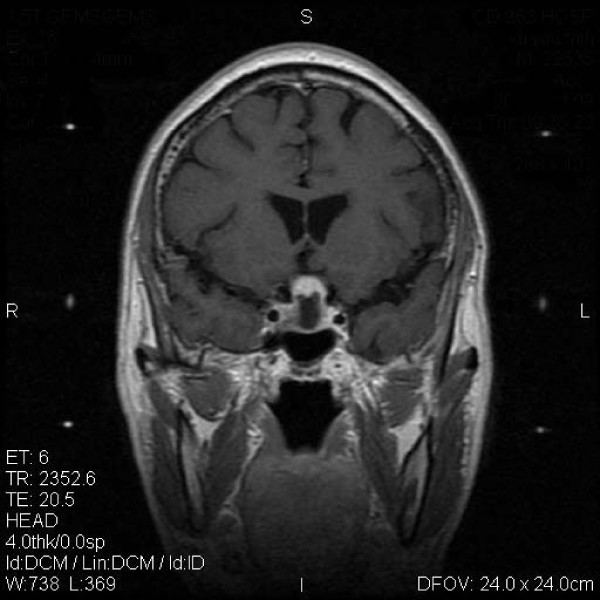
Typical MRI scan changes in prolactinomas adenoma. Coronal T1-weighted postcontrast MRI scan at left and right, obtained in Patient 2, a 27-year-old woman who presented with prolactinomas adenomas and amenorrhea-galactorrhea 4 years before undergoing MASEP GKRS. An asymmetrically enhancing mass lesion is seen in the sella turcia with extension to bilateral internal carotid artery. Patient 2's serum prolactin level was 183.7 ng/ml. The patient was treated with MASEP GKRS twice because of the huge volume of the mass. The second MASEP GKRS was performed 1 year after the first one. The tumor was treated separately with the lower and upper part in order to protect the optic chiasma. MRI was performed for treatment planning. 25 Gy defined to the 50% isodose line is used to cover the lower part of the pituitary tumor in the first treatment, and 18 Gy defined to the 50% isodose line is used to cover the upper part of the pituitary tumor in the second time.
